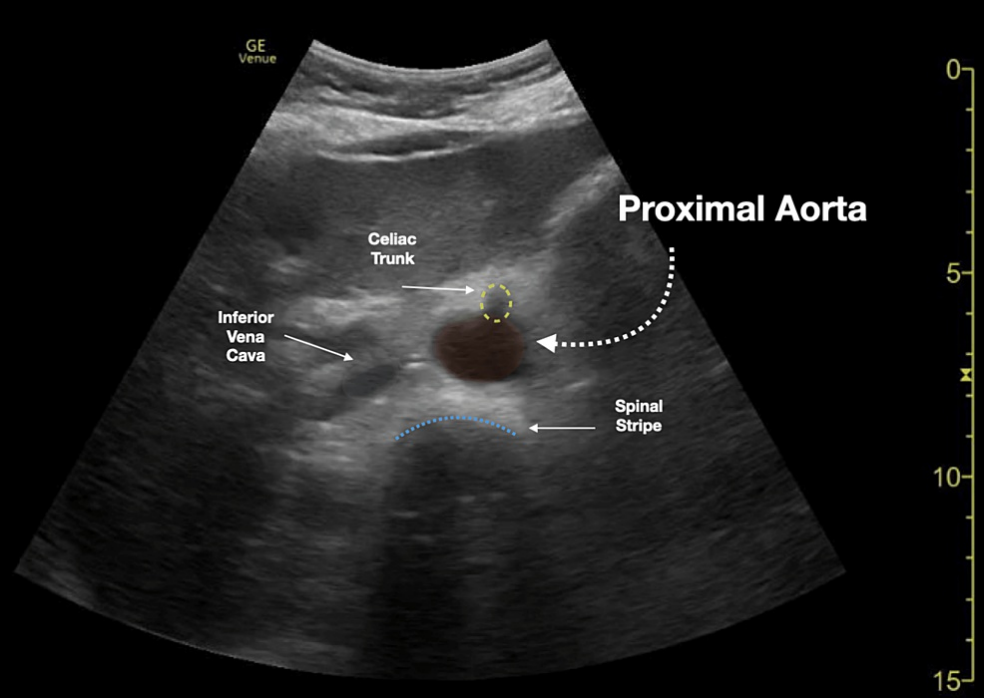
Proximal aorta ultrasound
